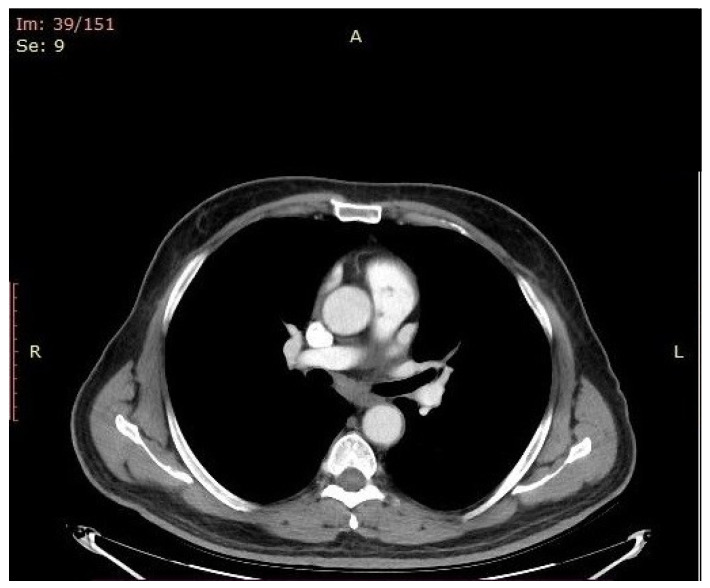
Pulmonary valve vegetations as depicted in CT scan images.
